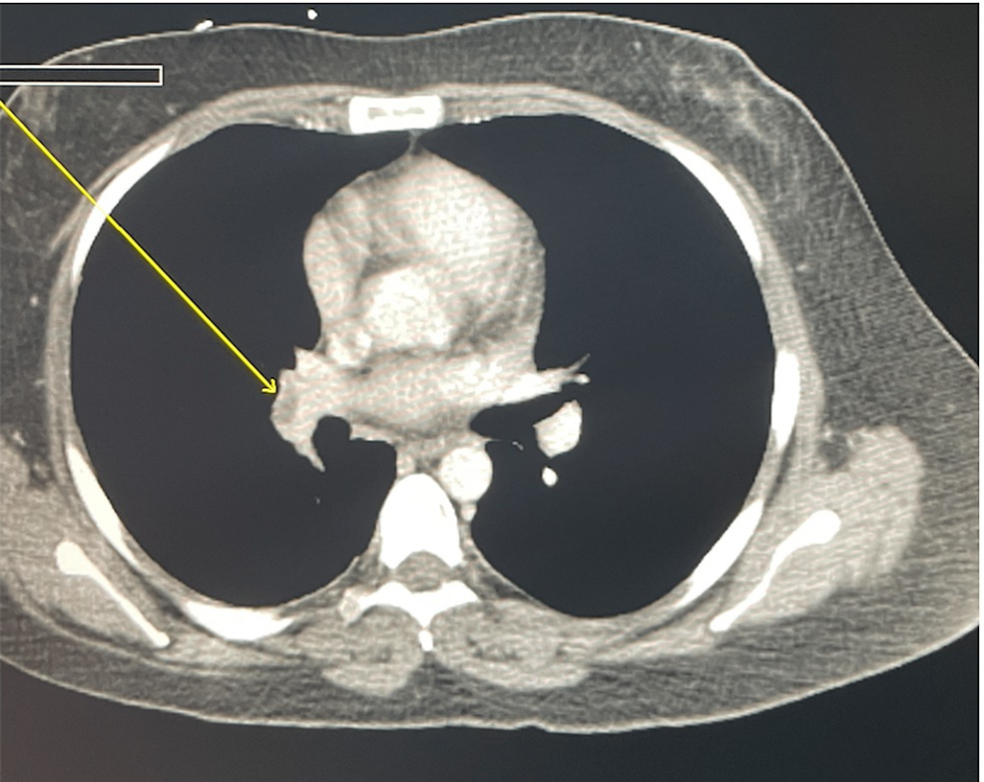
CT pulmonary angiogram: pulmonary emboli
